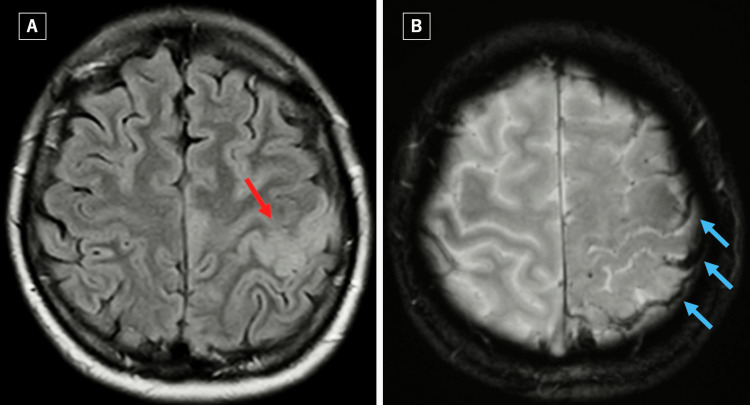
Fluid-attenuated inversion recovery (FLAIR) image shows cortical edema around the left central sulcus (red arrow) (A). T2*-weighted image shows cortical venous thrombi (blue arrows) (B).
